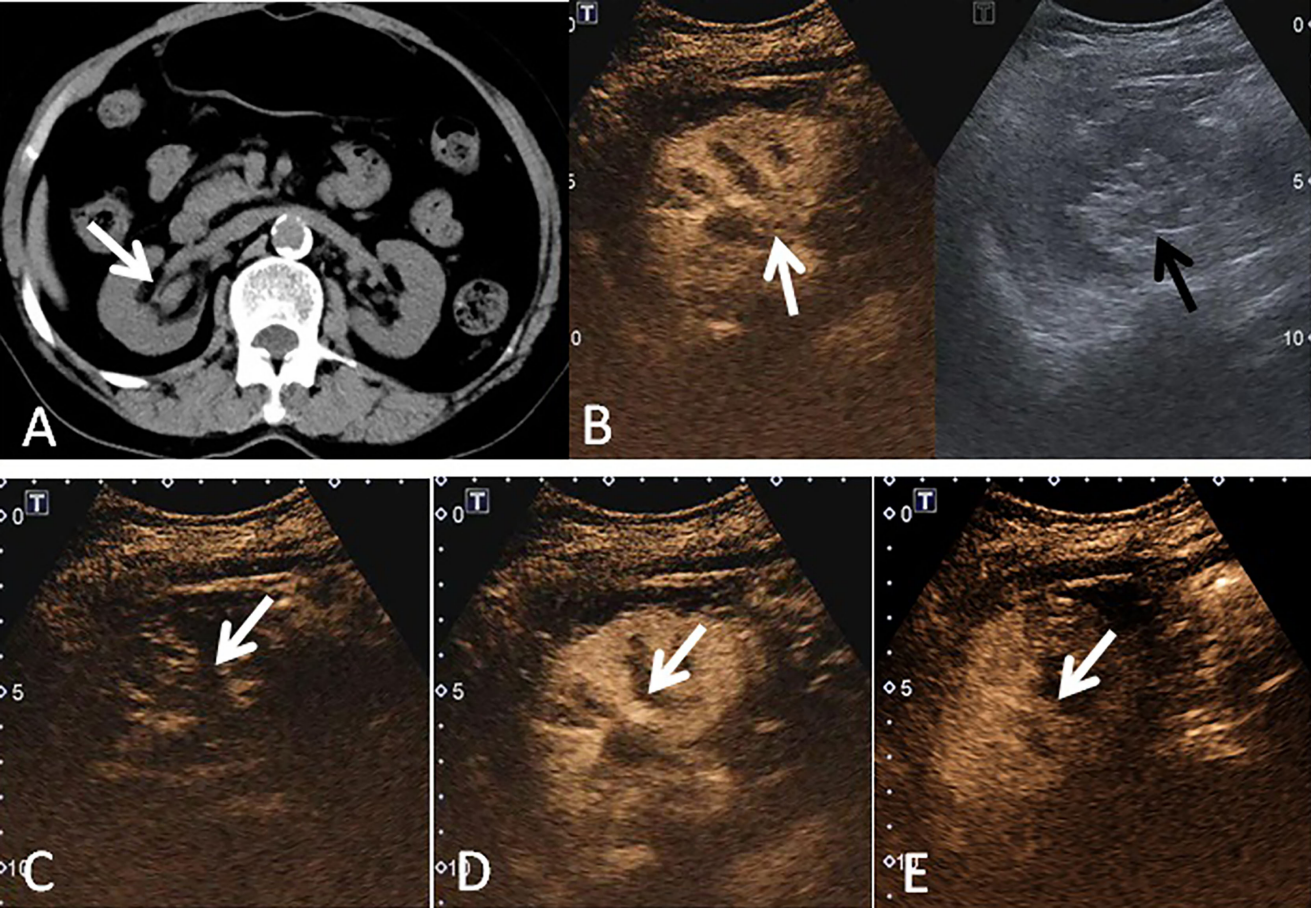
A 69-year-old woman had a UCRP in the right kidney with the size of 2.2 × 1.6 cm. (A) A suspicious renal pelvis mass (white arrow) was observed on non-enhanced CT scan. (B) According to the position displayed on CT, the simultaneous display mode of CUS (right) and CEUS (left) was performed, and CEUS demonstrated local contrast enhancement in the renal pelvis (white arrow). CUS showed a slight separation of the renal pelvis, but no lesion was observed (black arrow). (C) On CEUS imaging, it showed a slow-in pattern compared to the renal cortex (white arrow). (D) The enhancement intensity of the tumor was similar to that of the adjacent renal cortex (white arrow). (E) Compared to the renal cortex, the tumor showed a fast-out pattern (white arrow) on CEUS.
